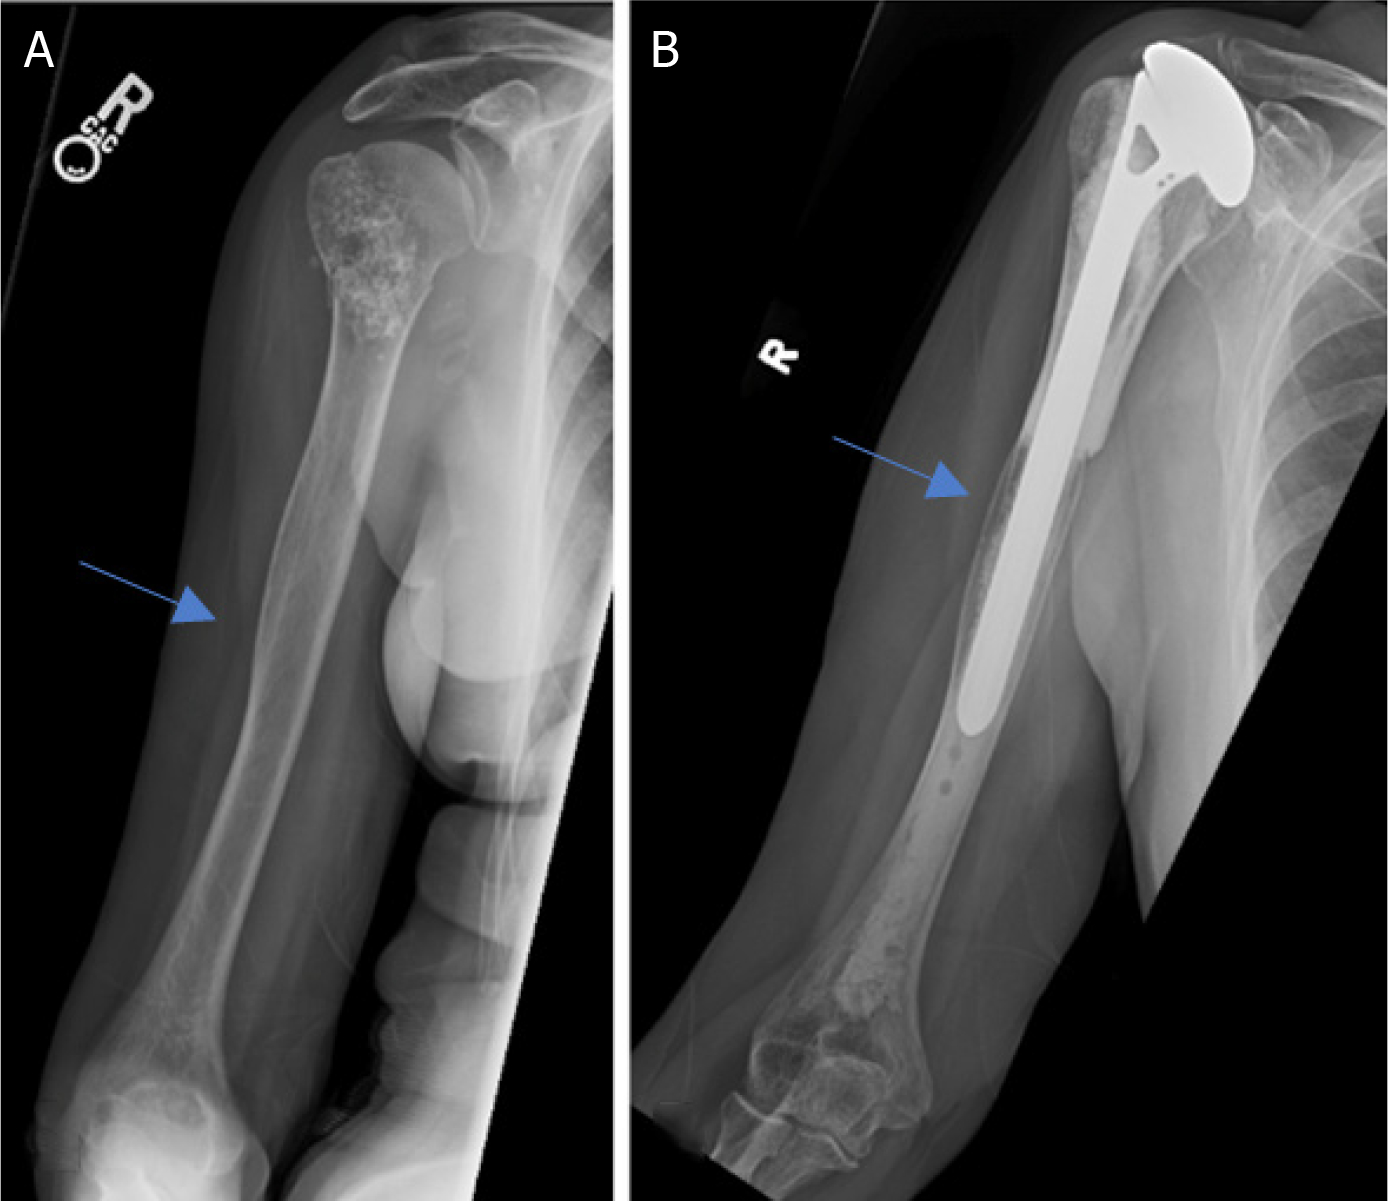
Anteroposterior radiographs of a 65-year-old male with: chondrosarcoma of the proximal humerus preoperatively (A); 4.5 years after wide excision and proximal humeral APC reconstruction (B). Blue arrow notes the junction between the allograft and native bone.
